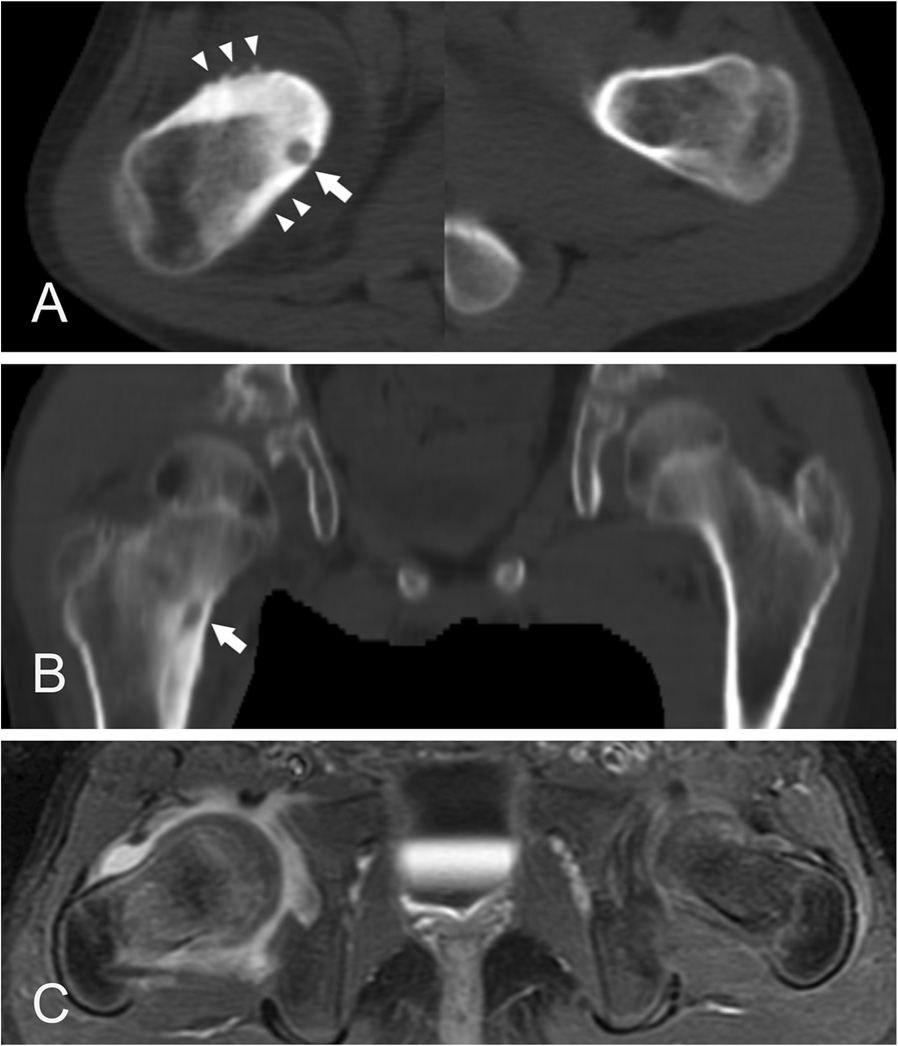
A 9.7-year-old girl with a juxta-articular osteoid osteoma of the proximal femur with involvement of the hip joint. (A) Axial CT image of the right femoral neck shows a prominent bony hypertrophy (arrowheads) around the nidus (arrow). (B) Coronal CT image demonstrates a nidus just above the lesser trochanter of the femur (arrow). Note the widening of the femoral neck and hypertrophy of the femoral head compared with the contralateral normal side. (C) Axial contrast-enhanced T1-weighted MR image with fat suppression shows synovial hypertrophy with effusion. The head-neck offset is not clear.
